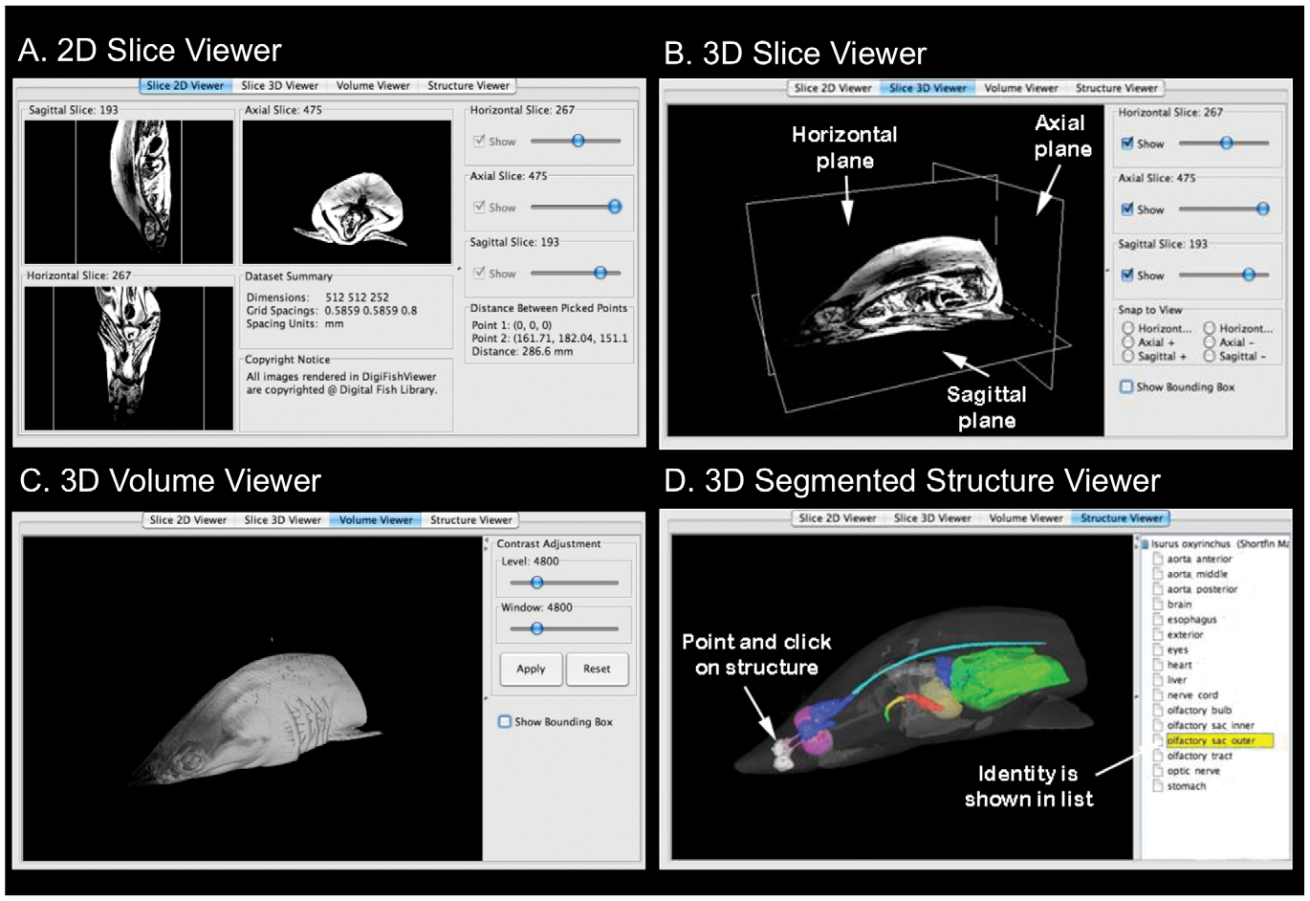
DigiFish Viewer with shortfin mako (*Isurus oxyrinchus*) MRI data. (A) 2D Slice Viewer displays slices in each orthogonal plane. Distances between points within slices can be measured. (B) 3D Slice Viewer displays an intersecting slice from each orthogonal plane in a single view that can be reoriented in 3D. (C) 3D Volume Viewer displays slice data as a 3D volume. Image contrast can be adjusted which also takes effect in the Slice Viewers. (D) 3D Segmented Structure Viewer displays 3D renderings of segmented structures. In this example, the skin (rendered partially transparent) and a selection of internal organs are shown. The olfactory sacs have been selected from the list of available structures and are highlighted (in white) in the viewer window.
